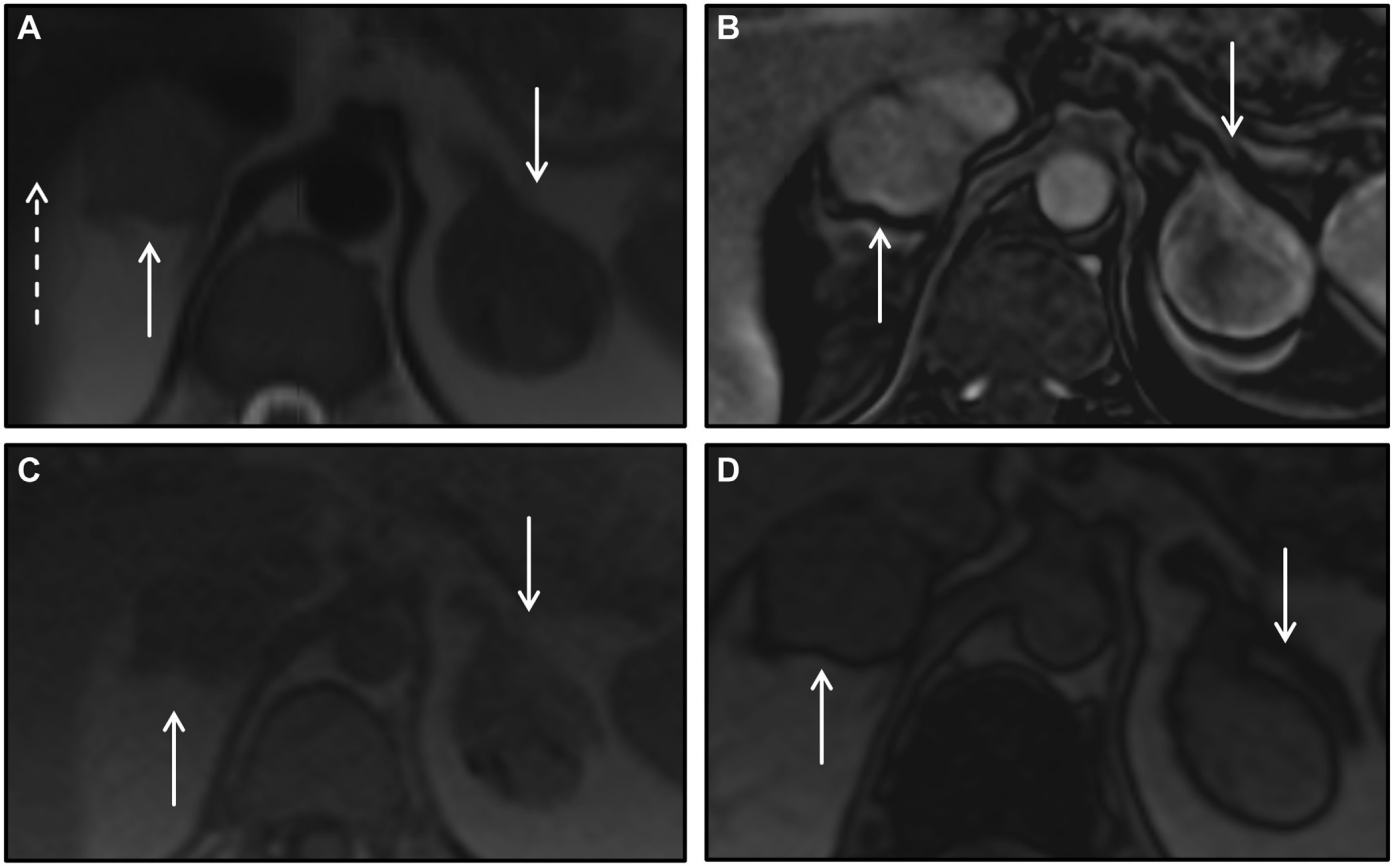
Bilateral adrenocortical neoplasms in multiple endocrine neoplasia type 1. (*A*) Axial T2 MRI image showing bilateral lesions with T2 intermediate signal, mildly above that of the adjacent liver (dashed arrow). (*B*) Axial post contrast image demonstrates heterogenous enhancement. (*C*-*D*) Axial in-phase (*C*) and opposed-phase (*D*) MRI images do not show any signal loss. Findings are not typical of adenomas. *CT* = computed tomography; *MRI* = magnetic resonance imaging.
